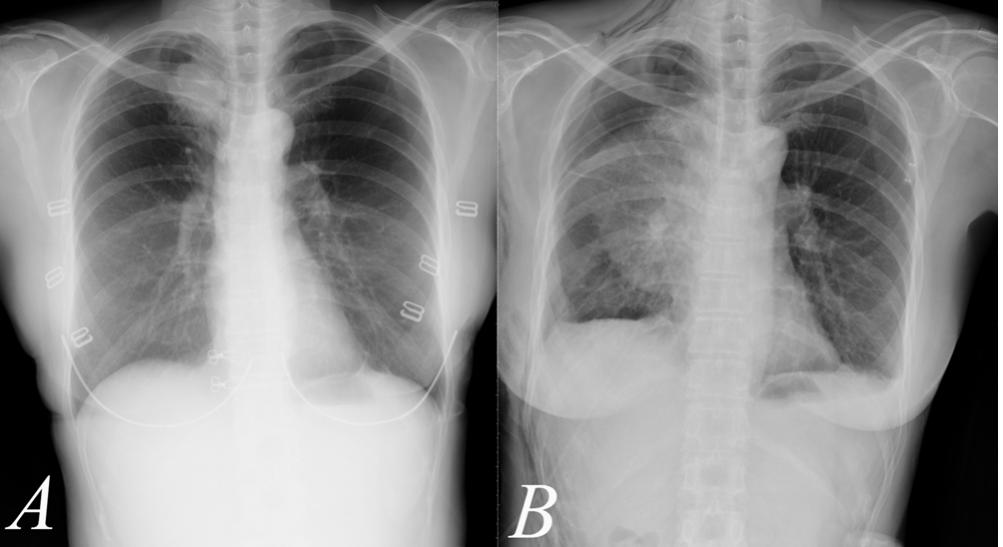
A. mass measuring around 3.5 cm in greatest diameterin right upper lung field. B. Follow-up chest radiograph showed a wedge-shaped opacity of large area in the middle lung field.
